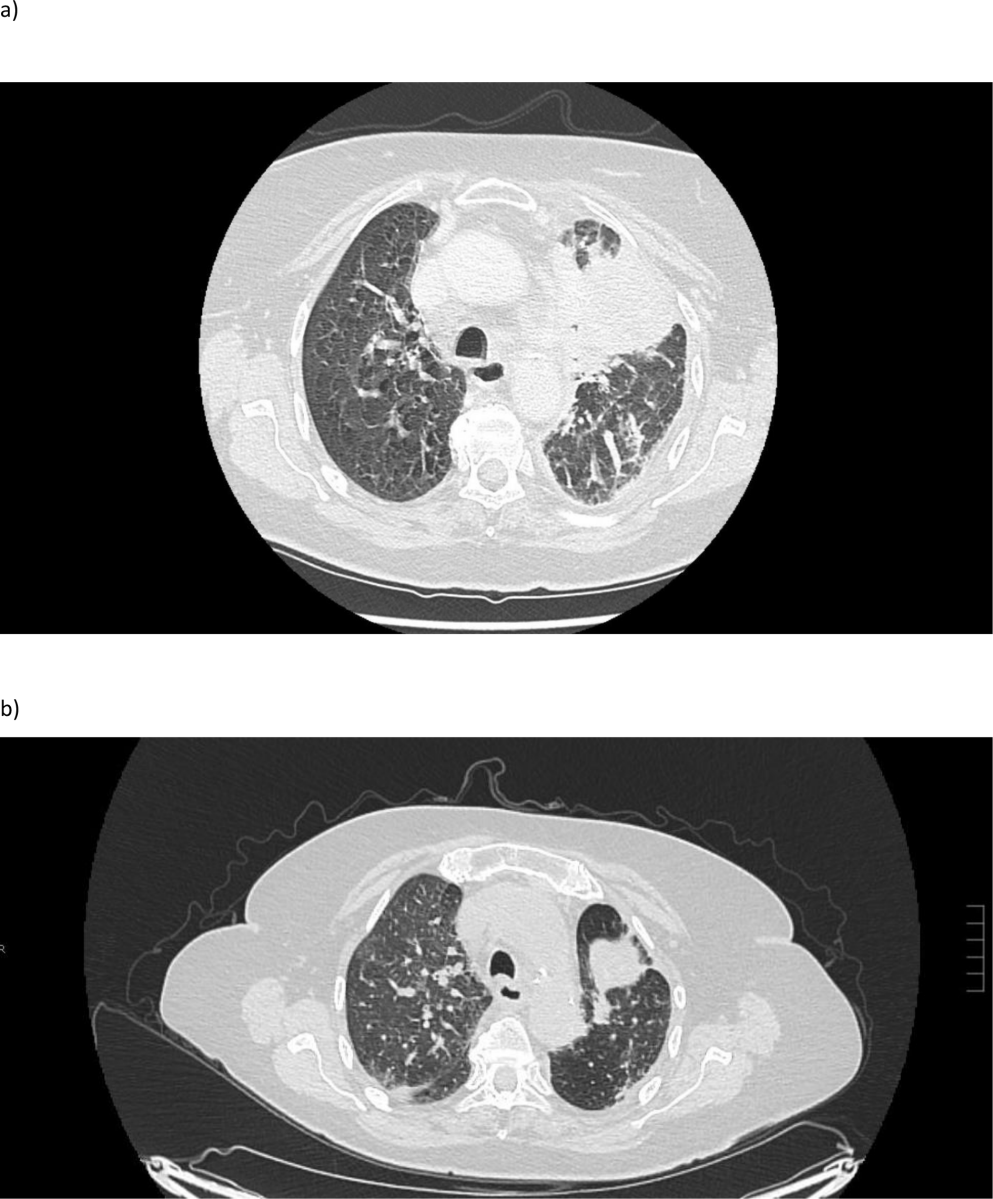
^18^F-FDG PET/CT scan at diagnosis before (a) and after treatment with osimertinib in combination with selpercatinib (b).
